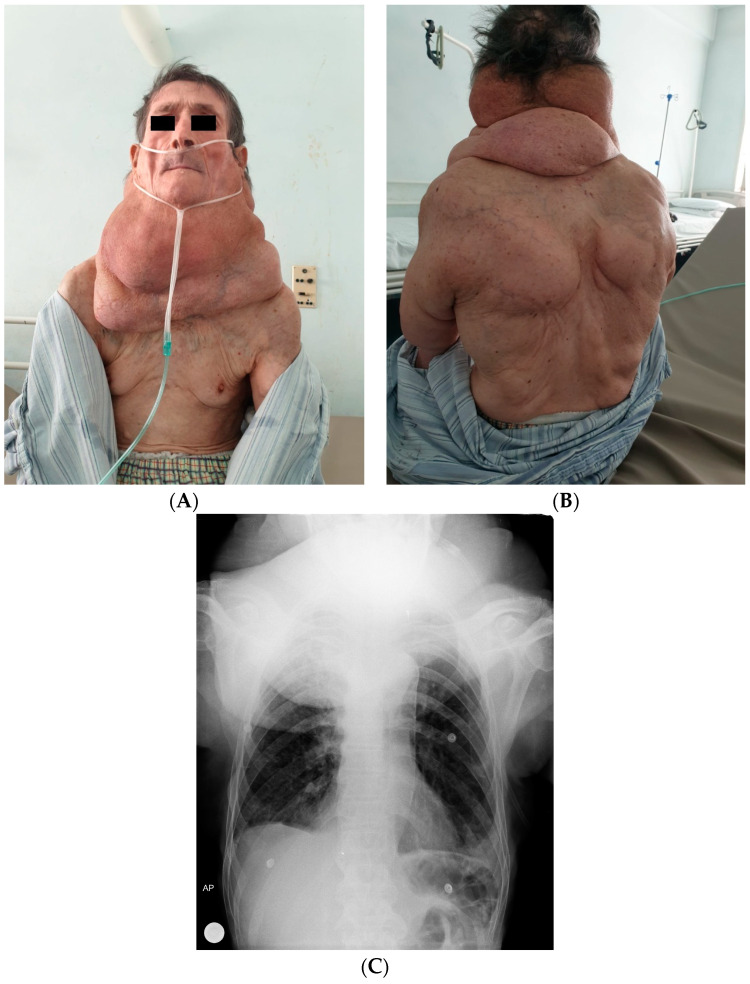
Large adenolipomatosis masses, in the cervical, thoracic, and arms regions: (A) front picture; (B) back picture; (C) X-ray of the thorax.
